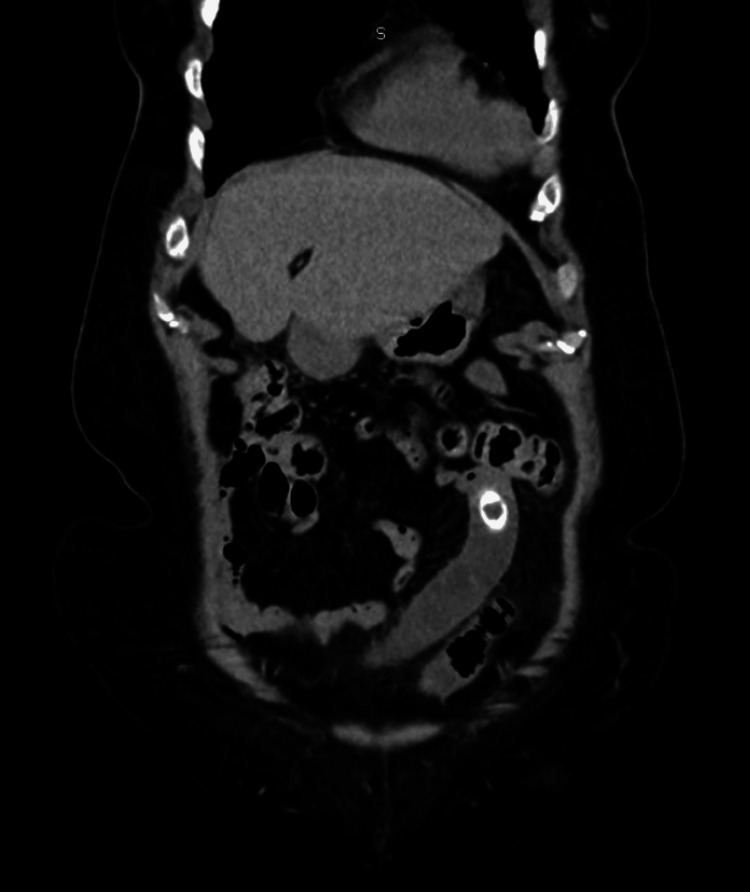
Coronal View of Abdominal CT Scan A hyperdense image consistent with a gallstone measuring 13.2 mm × 22.1 mm was observed, with dilation of the small bowel loops. CT: computed tomography
